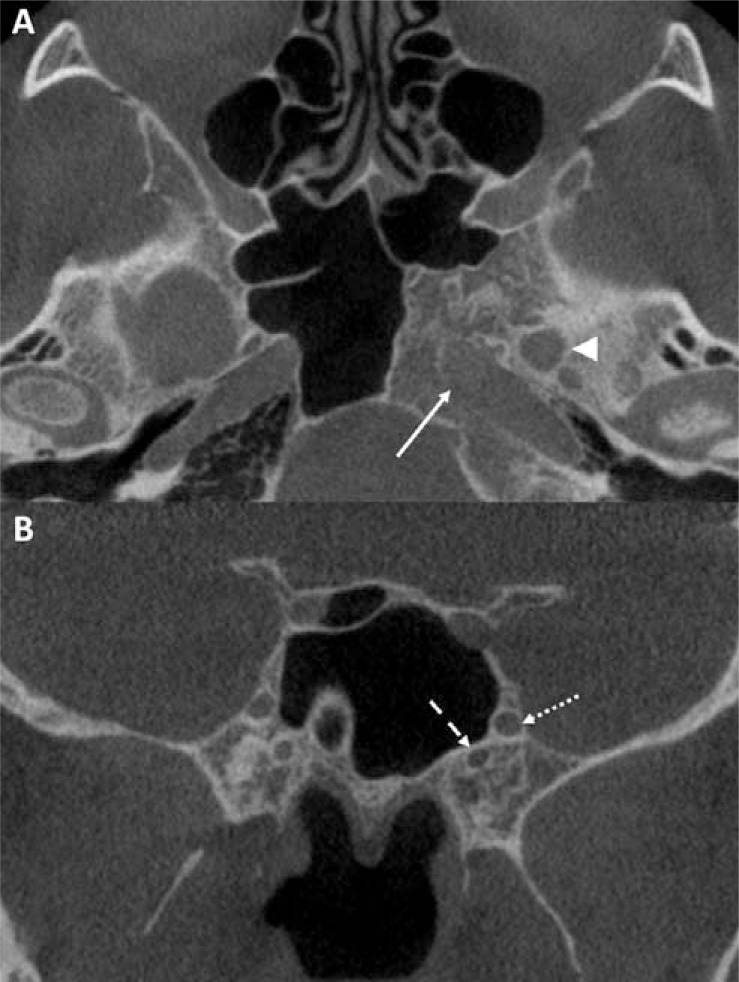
Examples of anatomical structures involved in arrested pneumatization of the sphenoid (APS). No effects on the surrounding structures were noted in (A) axial CBCT image with APS extension to the left foramen ovale (*arrowhead*) and the carotid canal (*solid arrow*) (male, 45 years), and (B) coronal CBCT image of left APS extension to the foramen rotundum (*dotted arrow*) and vidian canal (*dashed arrow*) (male, 48 years), note incidental dense bone island in the right sphenoid.
